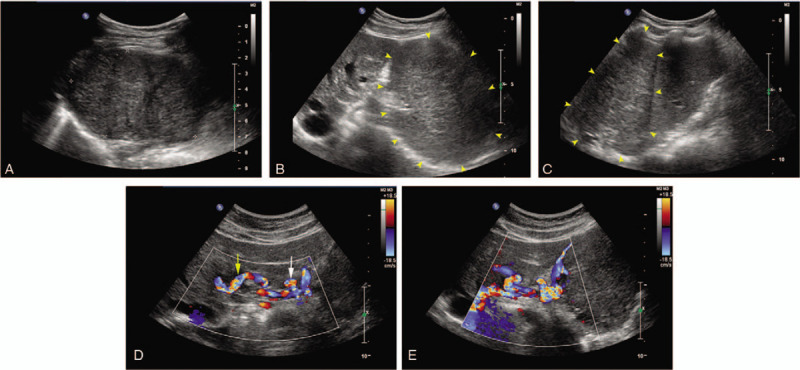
A. Gray scale ultrasound: a well-defined hypoechoic mass was found (calipers). B. Gray scale ultrasound: the mass (yellow arrow heads) was lateral to the left liver lobe. C. Gray scale ultrasound: the mass (yellow arrow heads) was close to the spleen, relative movement was present during respiration. D.E. The mass was highly vascularized by Doppler, a vascular pedicle was found between the mass (white arrow) and liver (yellow arrow).
